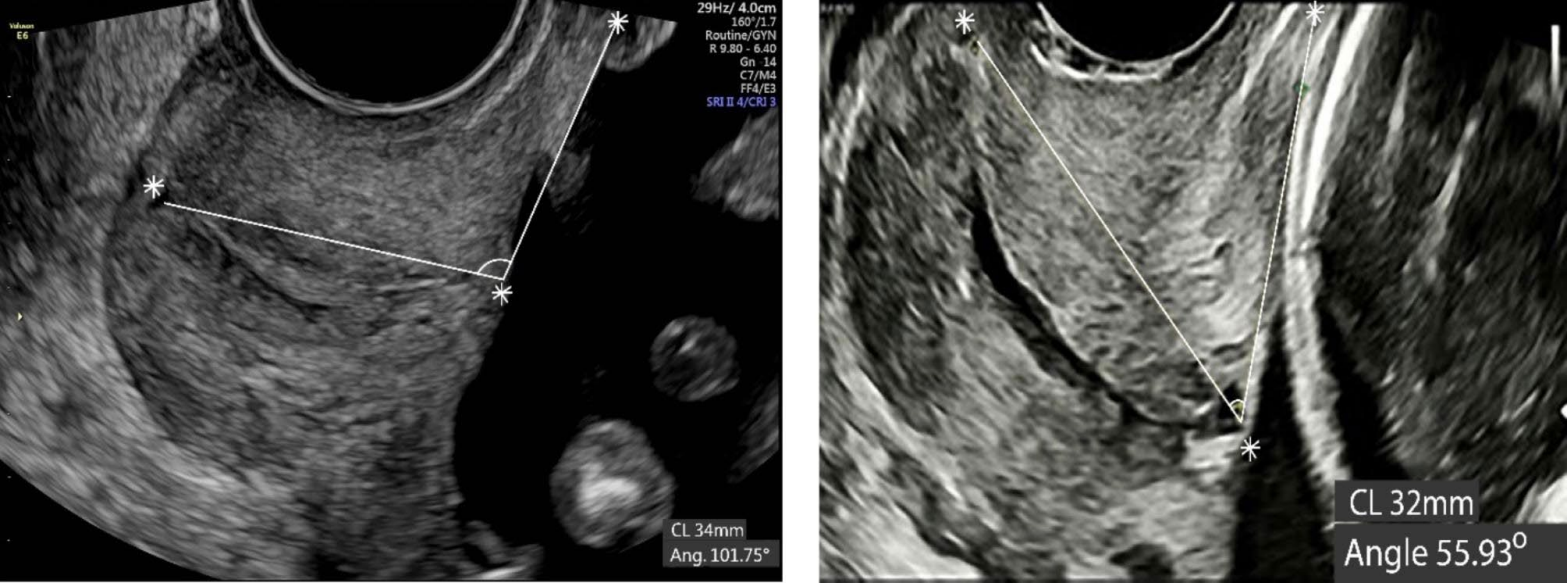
(a) GA 20^+ 0^ weeks, UCA 101.75^o^. (b) GA 22^+ 2^ weeks, UCA 55.93^o^. Transvaginal ultrasound measurement of the UCA. Measurement of the triangular segment between the lower uterine segment and the cervical canal
